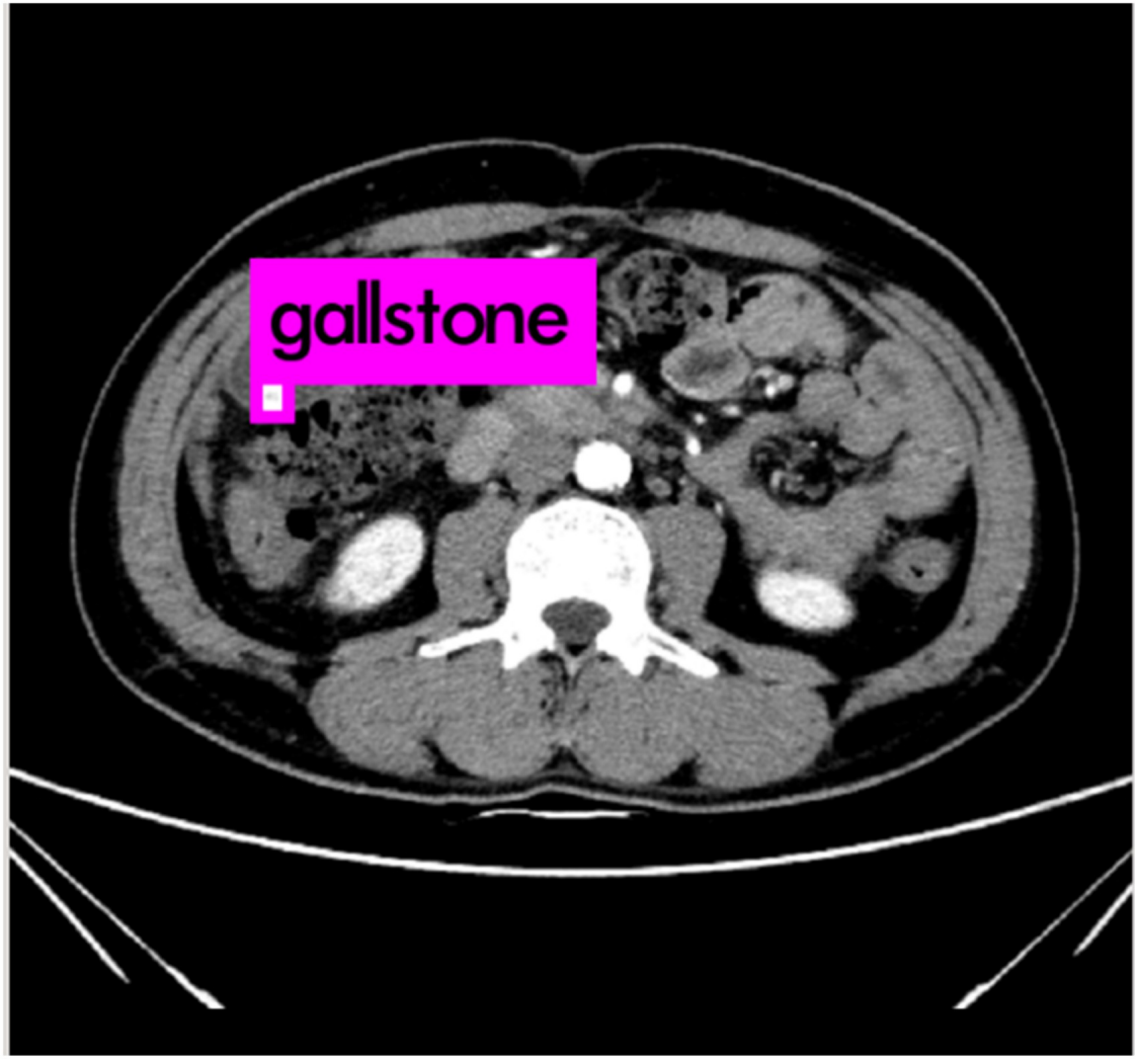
The CT image with the lesions discerned.
